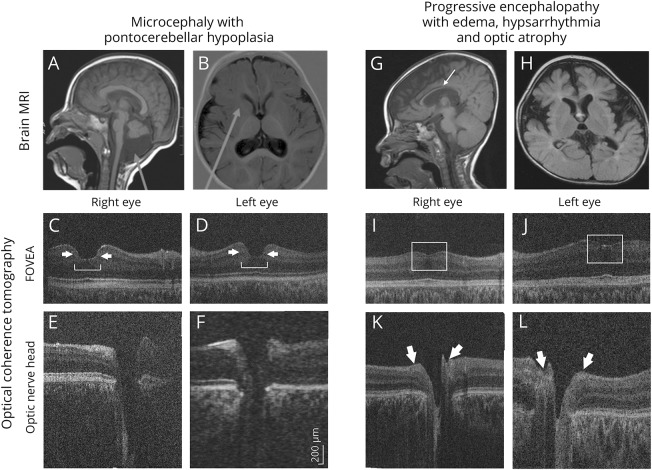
Patient 27 with microcephaly with pontine and cerebellar hypoplasia (MICPCH) and patient 22 with possible progressive encephalopathy with edema, hypsarrhythmia, and optic atrophy (PEHO) (A, B) MRI brain of patient 27 with MICPCH shows pontocerebellar hypoplasia, mega cisterna magna (A, arrow), and delayed myelination at the anterior limbs of the internal capsule (B, arrow). (C, D) Macular handheld (HH) optical coherence tomography (OCT) of the right and left eye displays a broadened fovea (bracket) with steep borders (arrows). (E, F) Optic nerve HH-OCT of the right and left eye with an enlarged cup-to-disc ratio of 0.8 at both eyes. (G, H) MRI brain of patient 22 with possible PEHO shows generalized brain atrophy, plagiocephaly, thin corpus callosum (arrow), and retarded myelination. (I, J) Macular HH-OCT of the right and left eye of patient 22 demonstrates diminished foveal depression due to foveal hypoplasia (open squares). (K, L) Optic nerve HH-OCT of the right and left eye of patient 22 with thinning of the peripapillary retinal nerve fiber layer (arrows).
